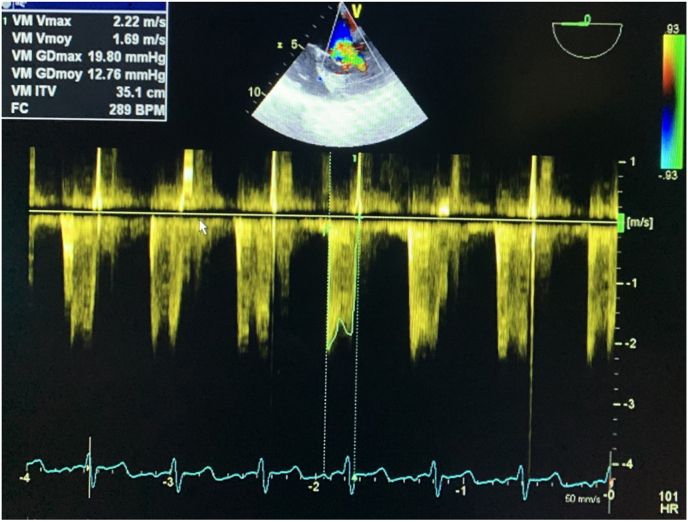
Trans esophageal echocardiography showed the same anomalies on The TTE a sub-mitral membrane, with a single sub-papillary muscle, an attachment defect of the mitral valves responsible of a mitral stenosis under the tight valve (the mean gradient at 12 mmHg) while the mitral surface was 2 cm ^2^ by planimetry on the TTE.
